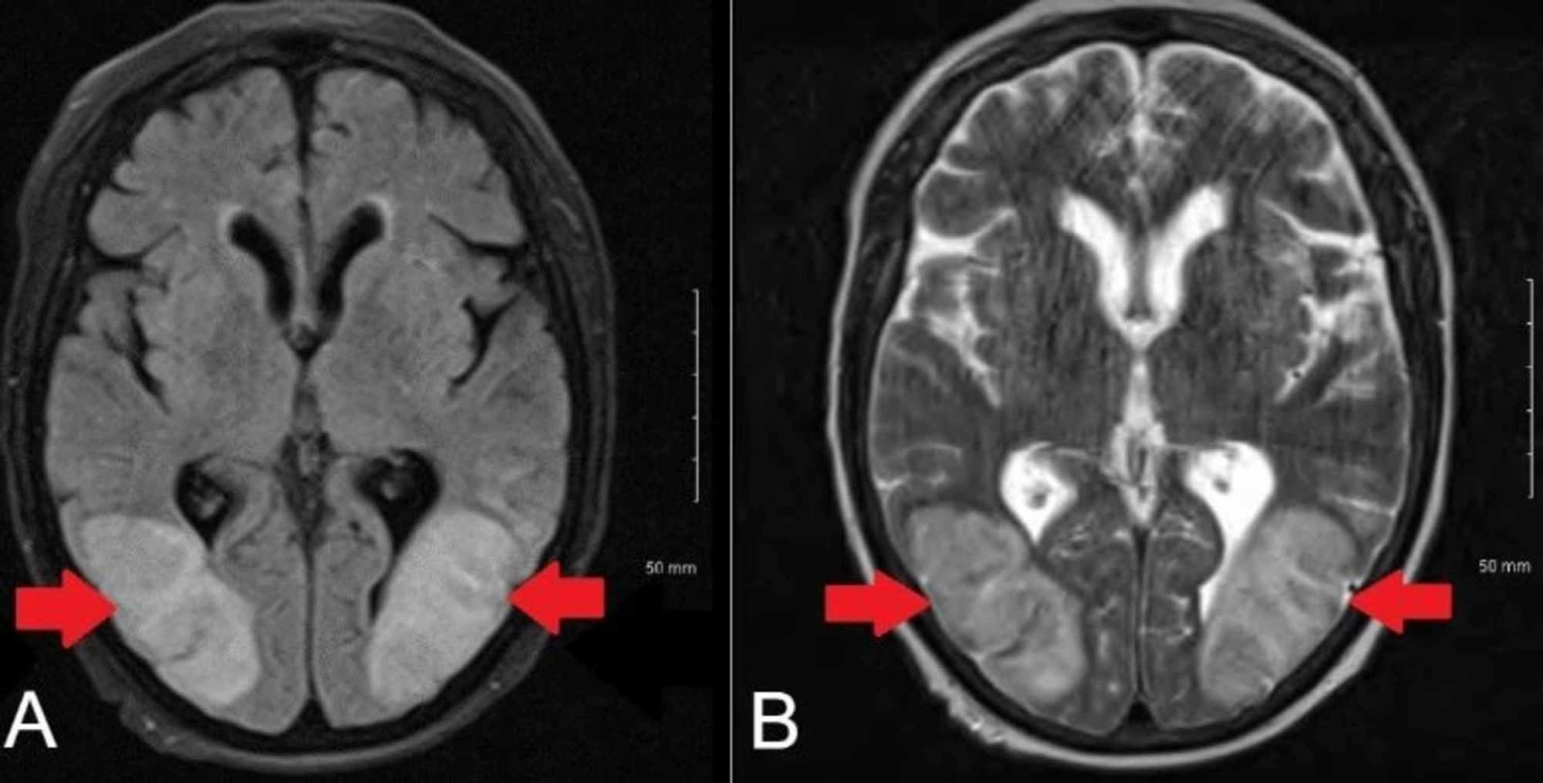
Magnetic resonance imaging axial view of the brain. (A) T1-weighted image revealing expansile region of lateral aspect of both occipital lobes. (B) Large relatively confluent expansile regions of T2 fluid-attenuated inversion recover (FLAIR) hyperintensity in the lateral aspects of both occipital lobes.
